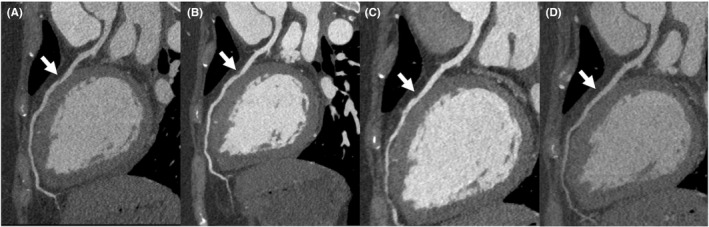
Coronary computed tomographic angiography images for follow‐up after PCI. Each image shows multiplanar reconstructed images of LAD, (A) 1 week, (B) 2 weeks, (C) 2 months, and (D) 10 months after PCI. The flap and coronary artery stenosis in the middle of the LAD (arrow) gradually disappeared
